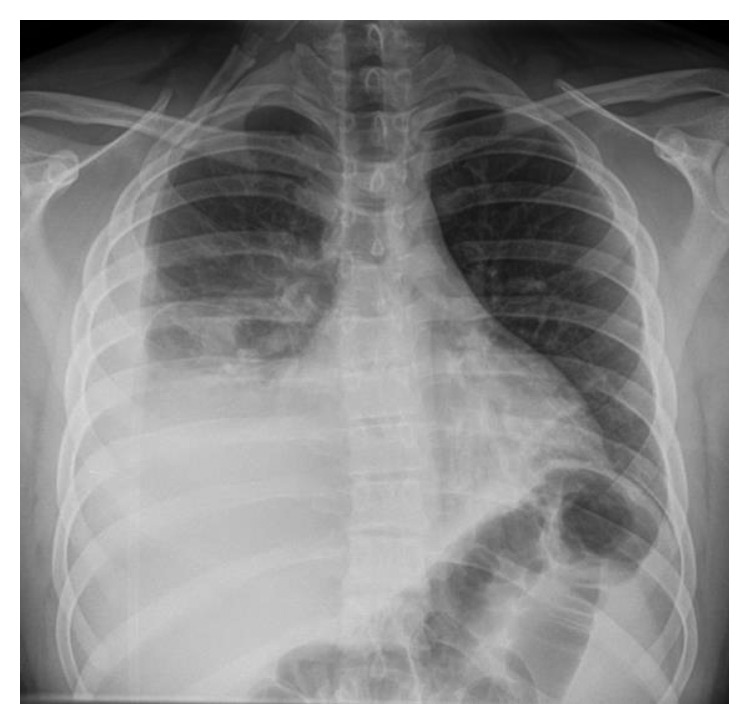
Second chest X-ray (after 48 hours). Right-sided severe pneumonia with parapneumonic effusion.
